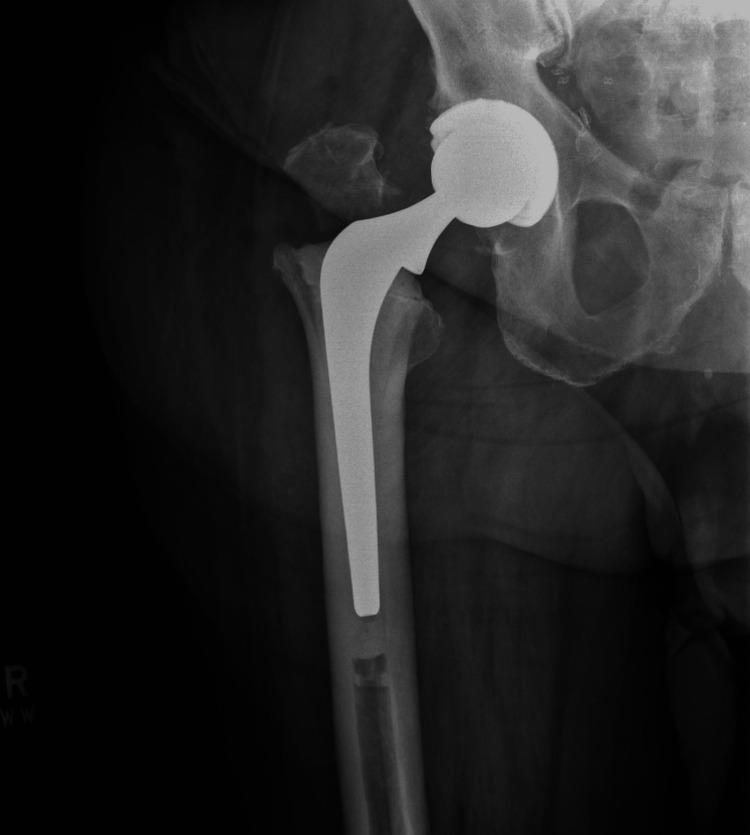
Postoperative cross-table lateral radiograph of the right hip Postoperative lateral radiograph confirming proper alignment of the cemented femoral stem and acetabular component without signs of dislocation or loosening.
